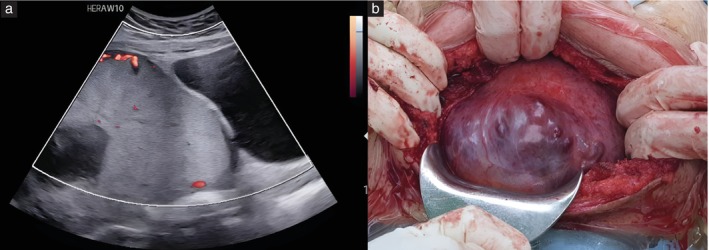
(a) Color Doppler ultrasound image in a patient with two previous Cesarean deliveries and anterior placenta previa with ultrasound features of lower uterine segment remodeling (placental bulge, myometrial thinning, loss of clear zone) and absence of uteroplacental vascular remodeling and serosal hypervascularity. (b) The intraoperative image demonstrates a wide area of uterine‐scar dehiscence, with the placenta visualized directly underneath the defect. Note absence of hypervascularity on the serosal surface. The placenta separated spontaneously from the uterine wall, confirming the diagnosis of uterine‐scar dehiscence with an underlying non‐adherent placenta.
